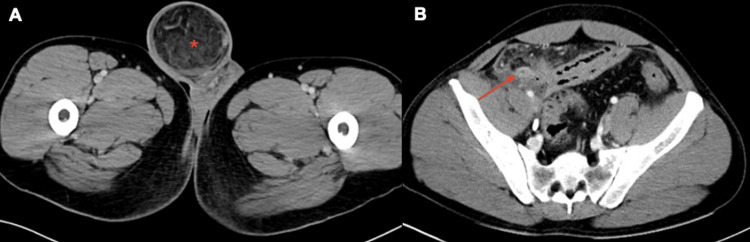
Axial (A and B) images of the abdomen and pelvis with intravenous contrast demonstrating a right-sided Amyand's hernia with perforated appendicitis There is radiographic evidence of an inflamed appendix (red arrow) protruding into the hernia sac (red asterisk). CT: computed tomography
